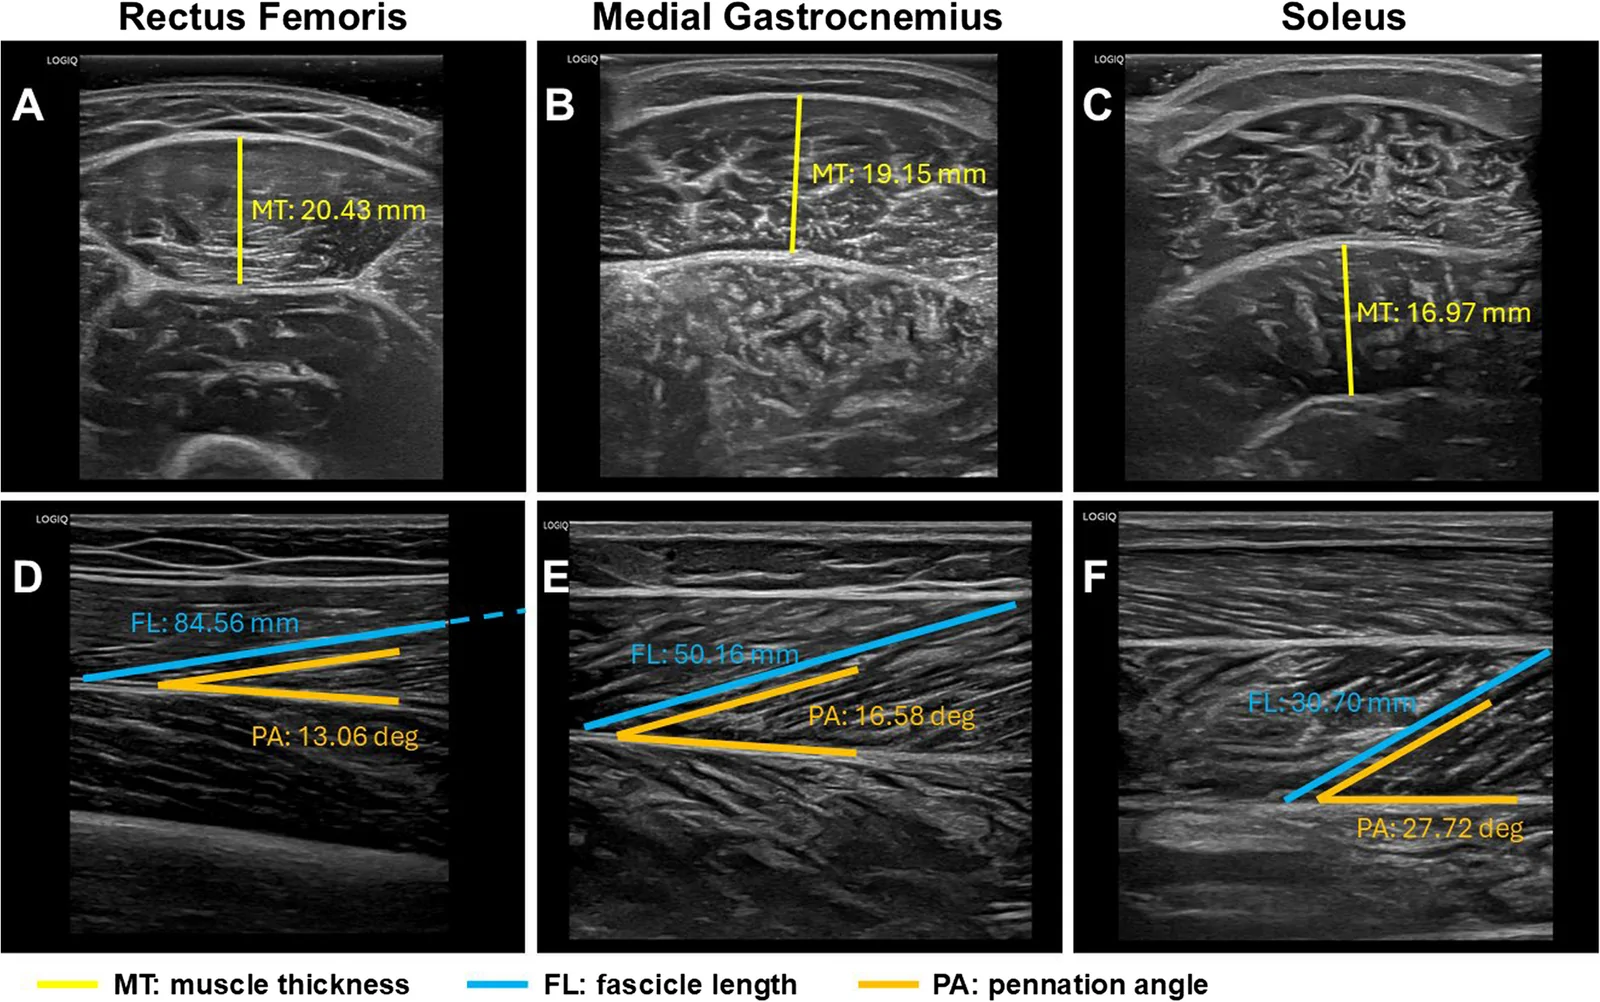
Representative B-mode ultrasonographic images of the RF, MG, and soleus muscles. Panels A–C show axial (transverse) images used for muscle thickness measurement; yellow lines indicate the distance between the superficial and deep aponeuroses, measured perpendicular to the fascicle plane. Panels D–F show longitudinal images used for fascicle length and pennation angle measurements; blue lines represent fascicle length traced from the superficial to the deep aponeurosis, and orange lines indicate the pennation angle between the muscle fascicle and the deep aponeurosis
